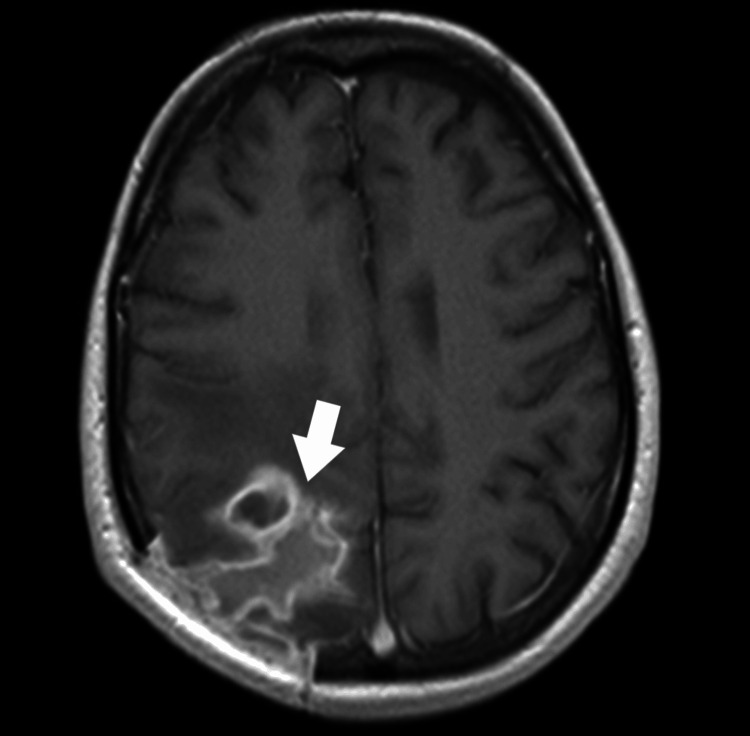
A follow-up MRI scan of the head revealed a postoperative lesion and possibly organizing suppuration: a top view (marked with a white arrow).
